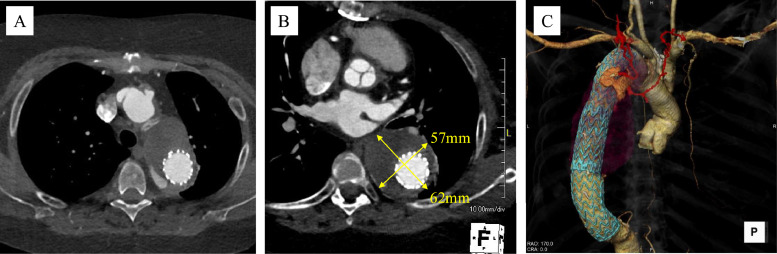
Computed tomography (CT) in Case 2. (A) CT revealed persistent endoleak in the proximal descending aorta. (B) The aneurysm gradually enlarged over a period of 3 years, reaching a maximum diameter of approximately 62 mm. (C) Feeding vessels included bilateral intercostal arteries originating from the thyrocervical arteries.
